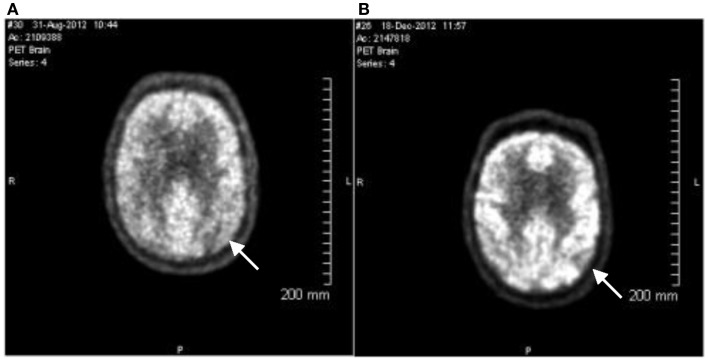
18-Fluorodeoxyglucose (18F) positron emission tomography images. PET images were evaluated in conjunction with CT images for both attenuation correction and anatomic fusion localization. Multiplanar PET images were obtained and evaluated as part of this study. (A) Image taken August 31st, 2012. Arrow indicates relative hypometabolism of right lateral parietal lobe. (B) Image taken December 18th, 2012. Findings are stable from the prior exam. Arrow indicates relative hypometabolism of right lateral parietal lobe. The pattern of hypometabolism involving the parietal and temporal lobes is stable and is consistent with the clinical diagnosis of probable Alzheimer’s disease.
